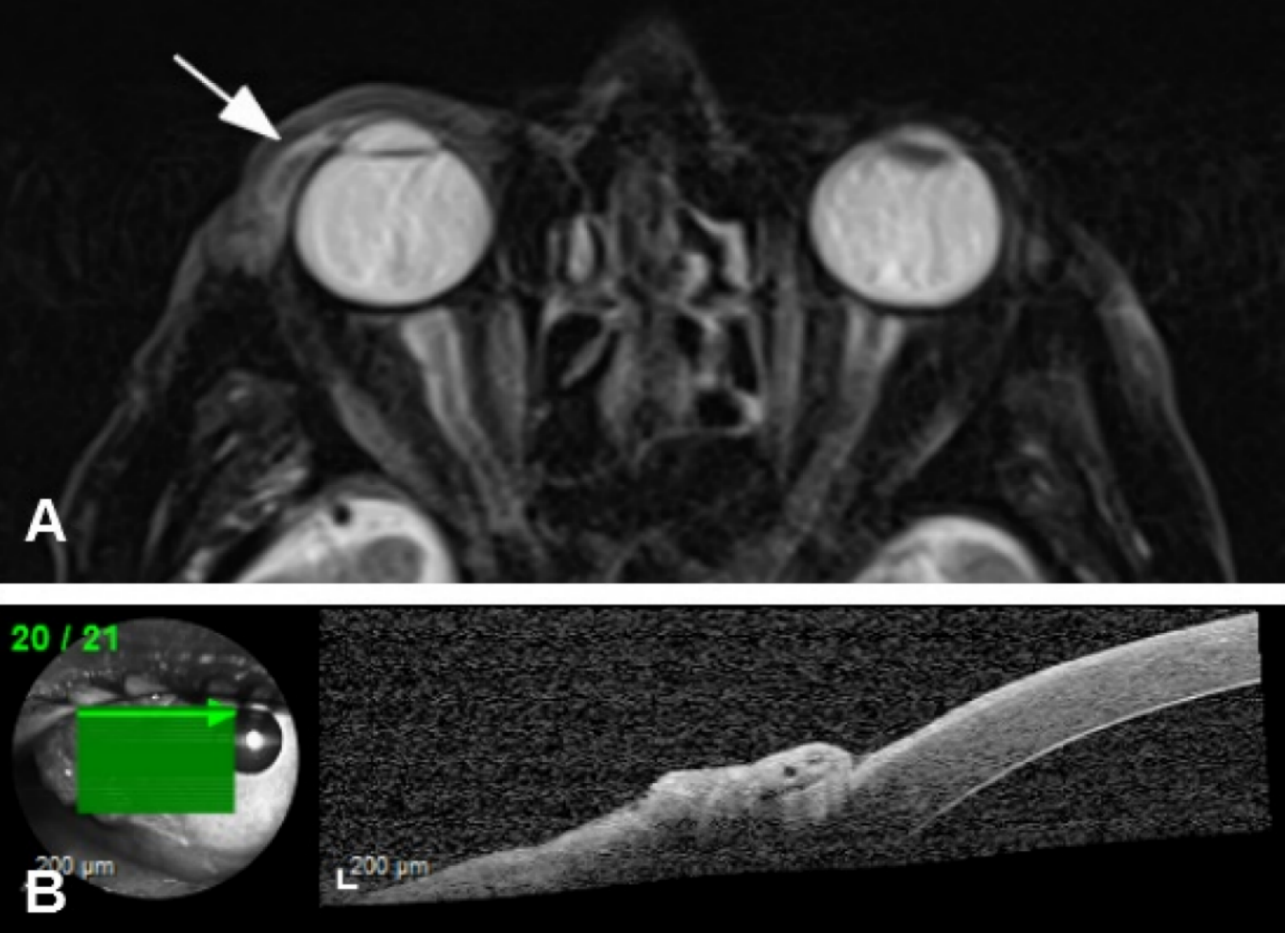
A) Magnetic Resonance Imaging showed lateral rectus muscle involvement. B) Anterior Segment – Optical Coherence Tomography (AS-OCT): a hyperreflective lesion involving conjunctival tissue and spread over corneal surface
